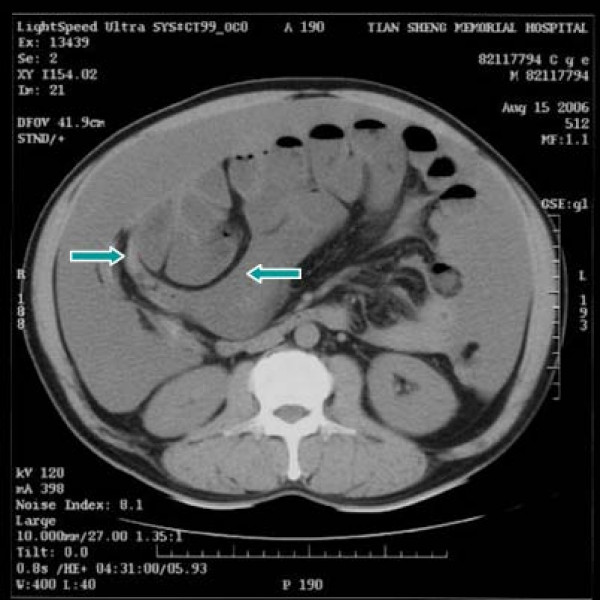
CT scan of abdomen showing pseudomyxoma peritonei with mucin septations (arrows).
